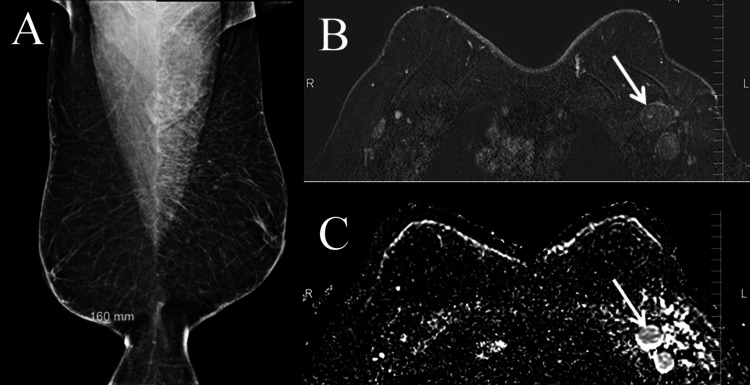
A 48-year-old male who presented with axillary swelling for nine months. No significant findings in blood analysis. Chest and abdominal CT only revealed suspicious left axillary lymph nodes. Biopsy of the lymph nodes identified metastatic invasive ductal carcinoma. Subsequent mammograms, ultrasound, breast MRI, and PET CT revealed large fatty gynecomastia with no breast lesions except positive axillary lymph nodes. (A) Bilateral mammograms in mediolateral oblique view. (B) Breast MRI contrast-enhanced subtraction view. (C) PET CT.
